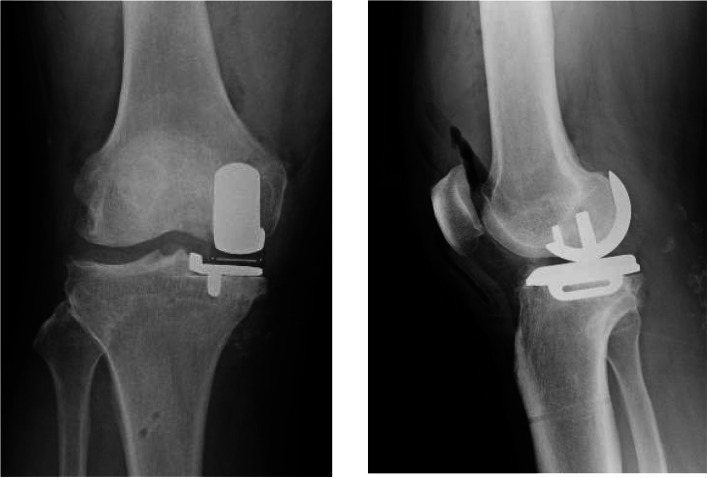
Case 2 radiographs. Postoperative plain radiographs of the left knee
